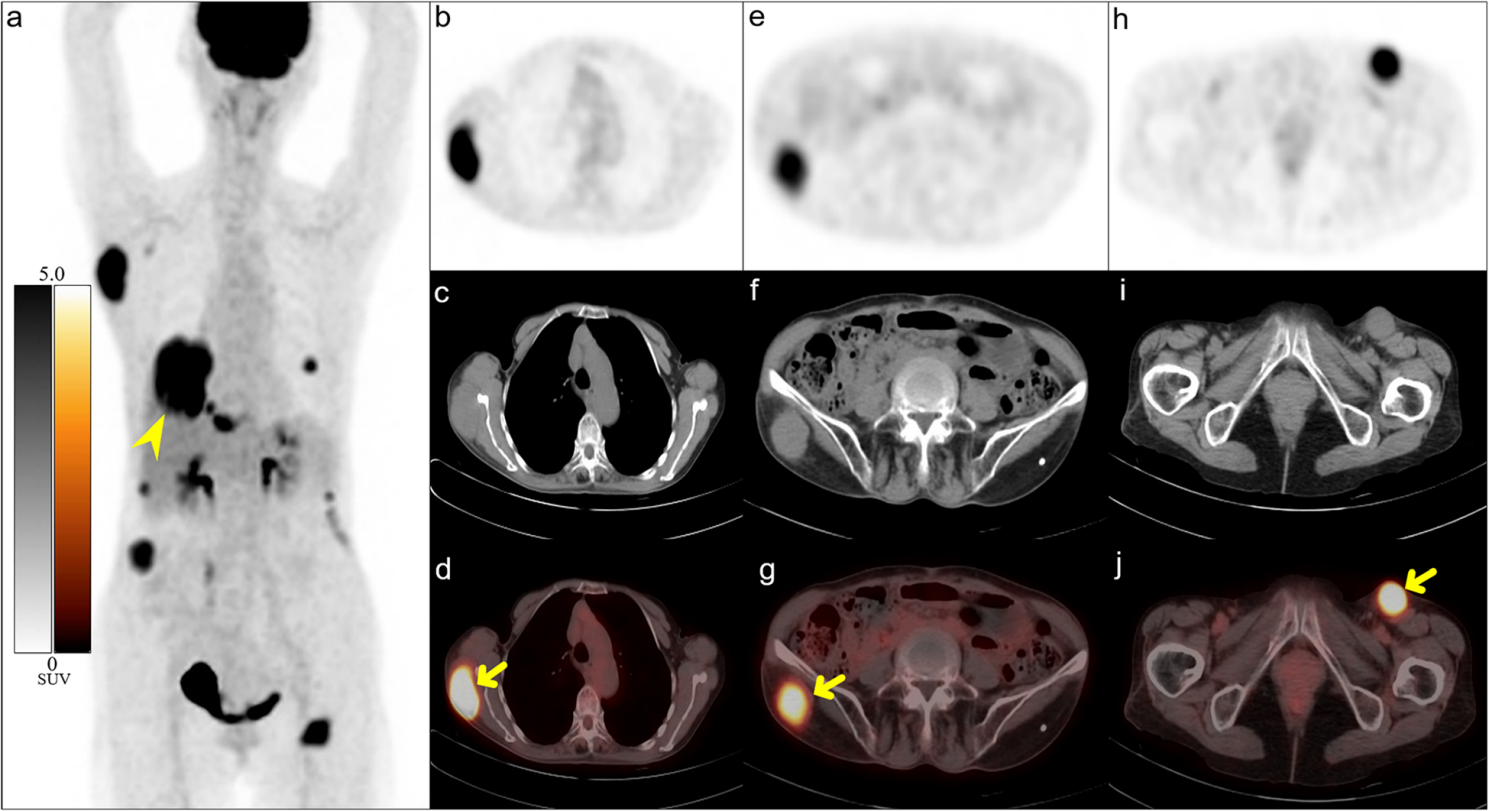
STM is the only manifestation of a small cell lung cancer. A 74-year-old woman presented with a 1-month history of a subcutaneous mass on the right side of her waist, which was confirmed as metastatic small cell carcinoma on biopsy. MIP (a) of ^18^F-FDG PET/CT showed a soft-tissue mass in the lower lobe of the right lung (arrowheads), with elevated FDG uptake (SUVmax = 8.4). MIP (a), chest axial images (b-d), and pelvis axial images (e-j) revealed multiple nodules and masses throughout subcutaneous tissue and skeletal muscle (short arrows) with increased FDG uptake (SUVmax = 7.5). Subsequently, lung biopsy confirmed small cell lung cancer of the right lung. After 11 months of palliative chemotherapy, the patient died of respiratory failure
